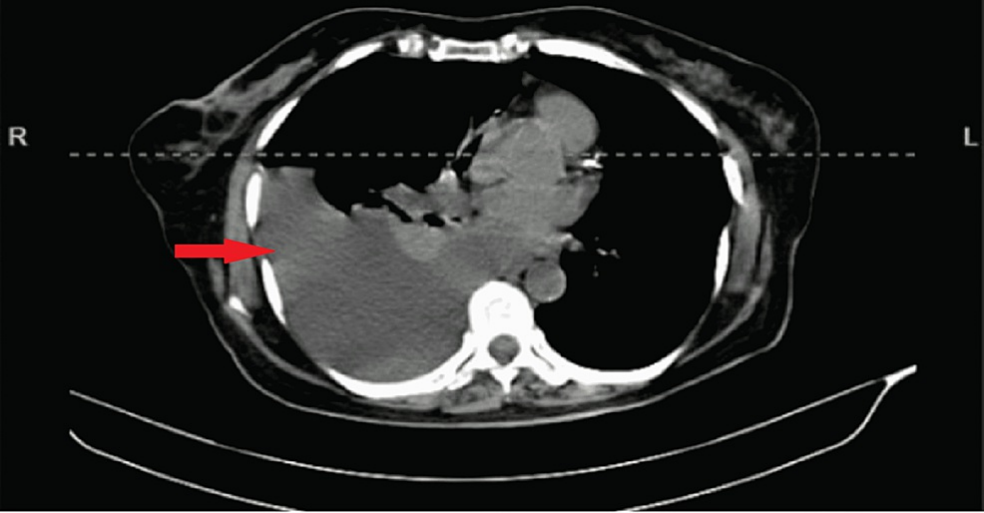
CT scan revealed a massive pleural effusion on the right side CT: Computed tomography
